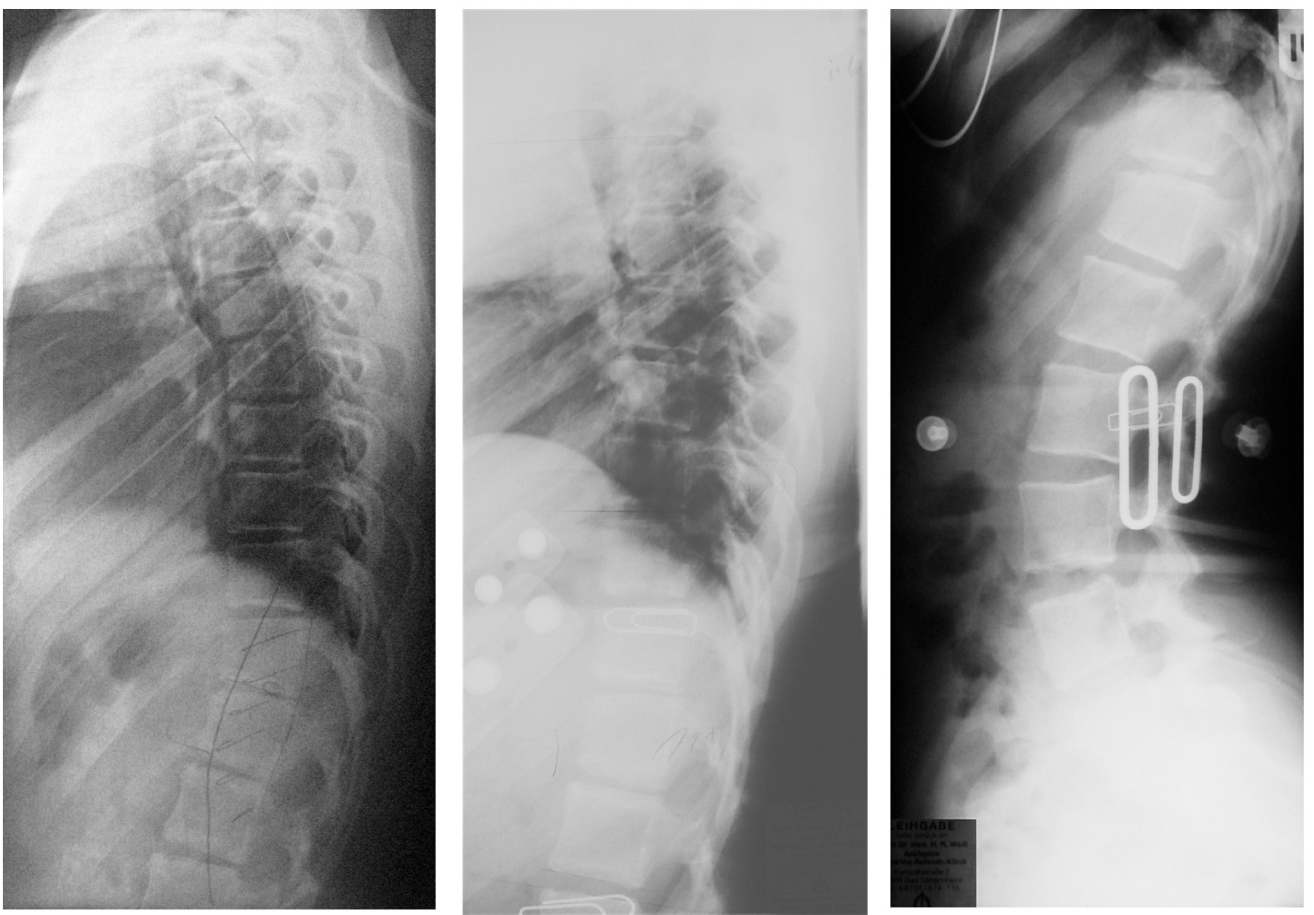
Lateral X-rays of a patient with thoracolumbar kyphosis. Left: lateral X-ray without brace, middle: lateral X-ray in the kyphologic™ brace model for thoracolumbar kyphosis without any significant effect. Right: In the physio-logic™ brace at least the lumbar lordosis is restored giving the sagittal view a more physiological pattern.
